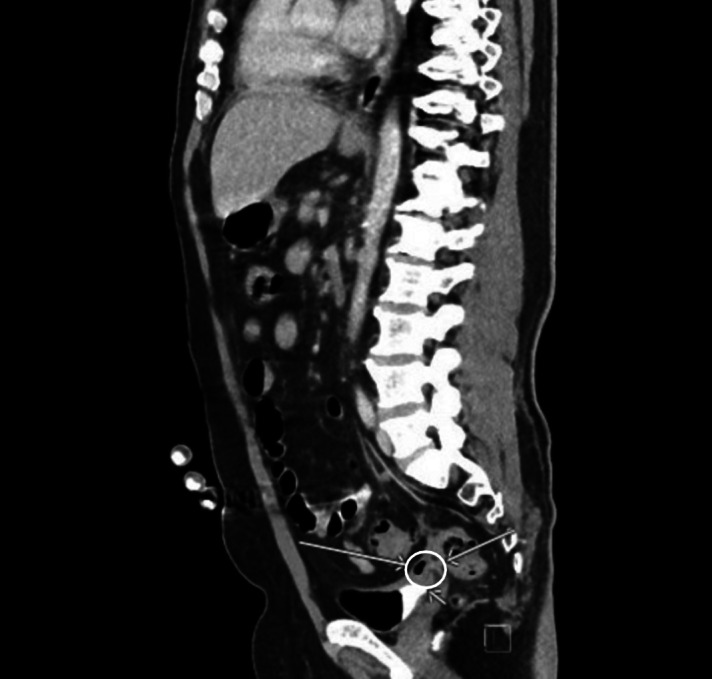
A sagittal view of computed tomography of the abdomen and pelvis demonstrating sigmoid diverticulitis with a colovesicular fistula (circle, arrows).
